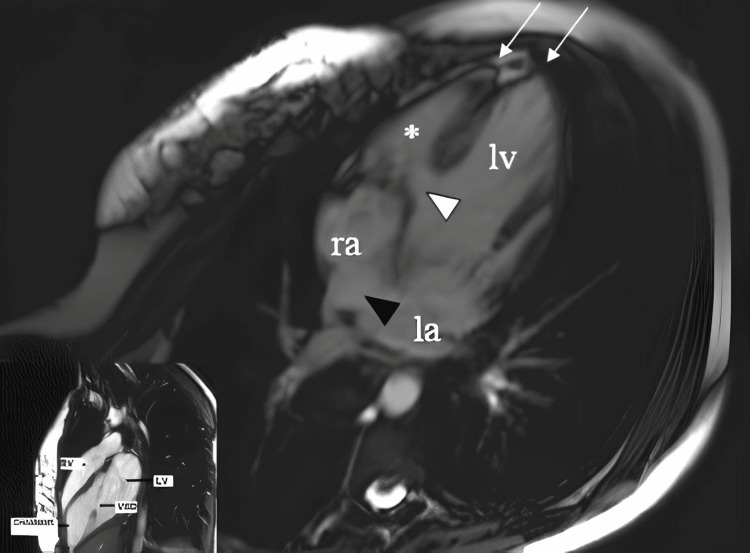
Cardiac MRI showing atrial septal defect (black arrow) and ventricular septal defect (white arrow). The image also reveals a bifid left ventricular apex, suggesting a rare structural abnormality contributing to the patient’s condition.
